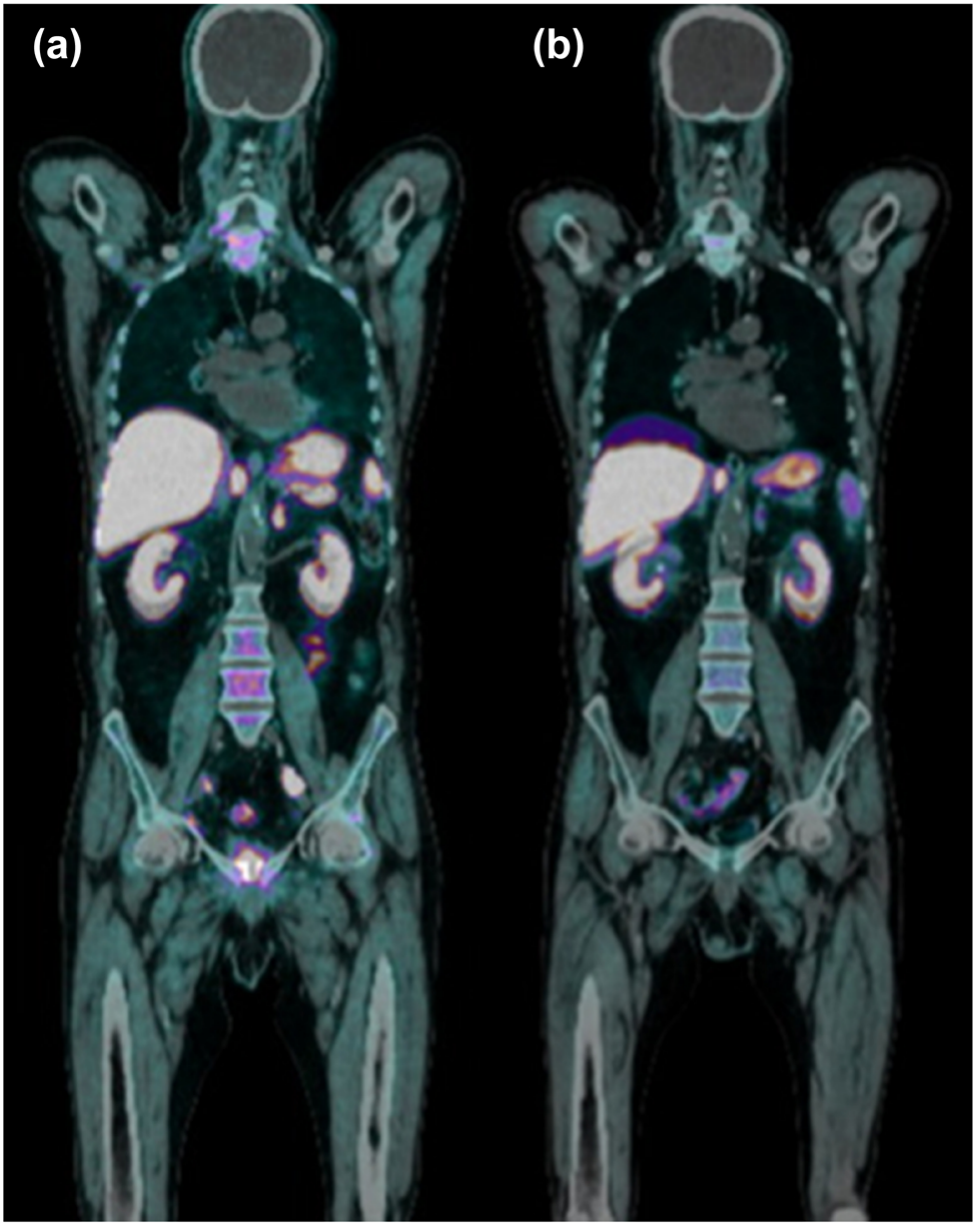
Choline PET-CT detects a left external iliac lymphadenopathy and a further capture of the tracer at the level of other left external iliac lymph nodes and inguinal lymph nodes bilaterally (a). Choline PET-CT performed after cystectomy and lymphadenectomy where previously highlighted lymphadenopathies are no longer visible (b).
